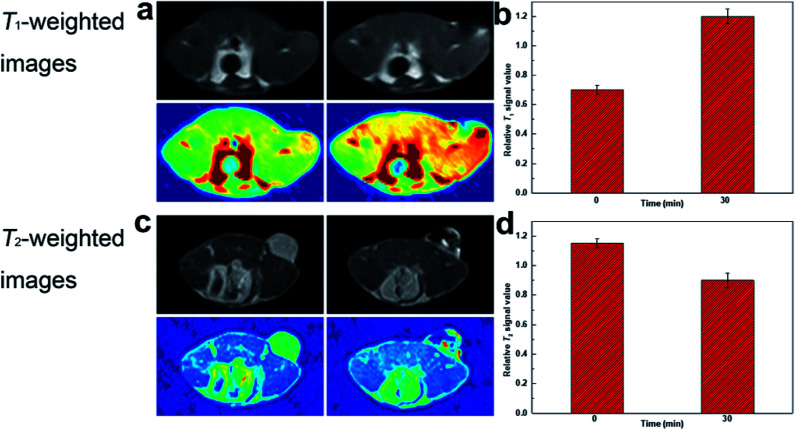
(a) *T*_1_-weighted and (c) *T*_2_-weighted MR images of tumor injected with DOTA(Gd)-Fe@Fe_3_O_4_ NPs; (b) and (d) relative signal intensity for *T*_1_- and *T*_2_-weighted MR images in tumor.
